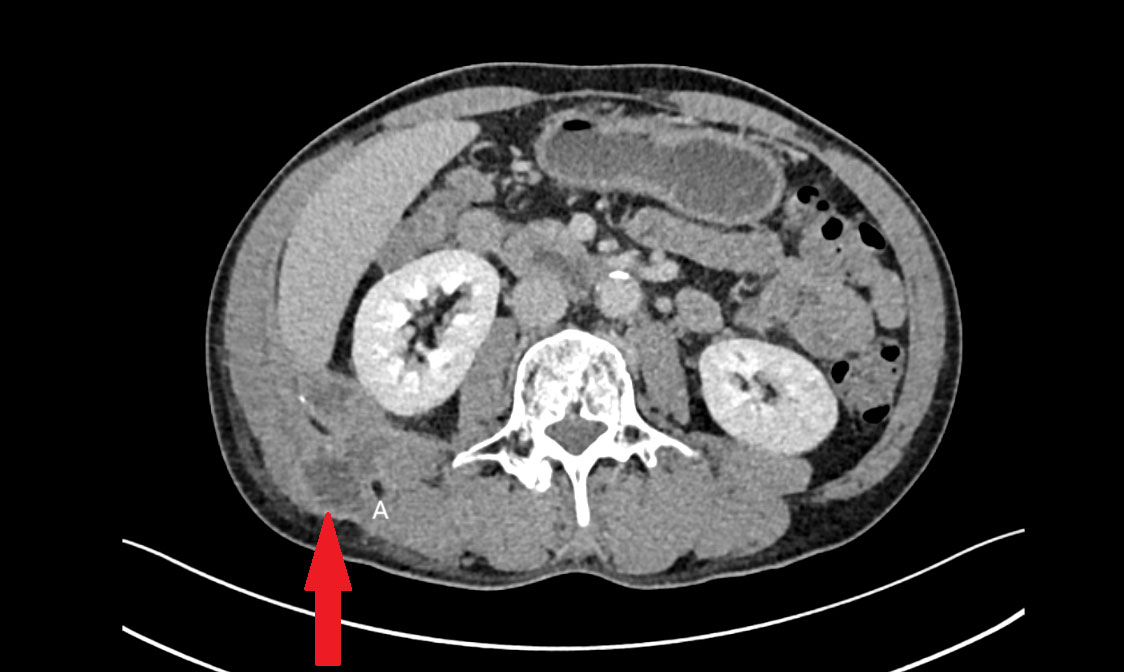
Contrast-enhanced CT showing the abscess in the abdominal wall. The abscess is indicated by the red arrow.
